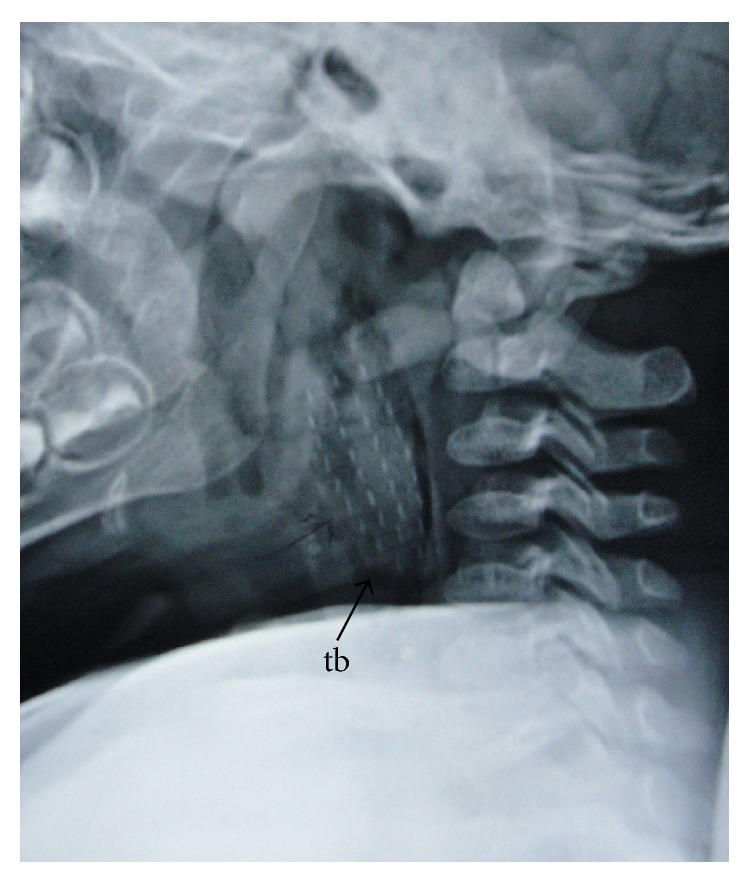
X-ray of the neck showing the head of the toothbrush (tb) with the bristles (dotted lines) in the retropharyngeal space extending from the level of C1 to C5 vertebrae.
